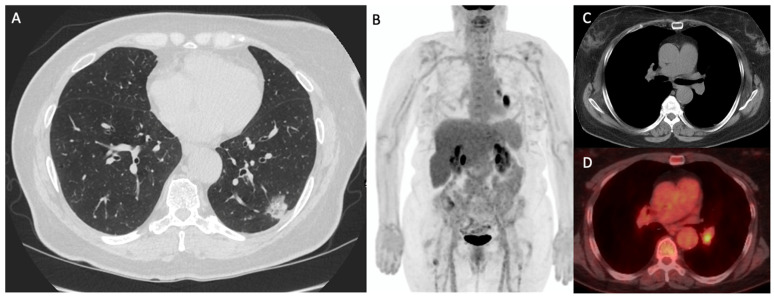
Demonstrates a 62-year-old woman with biopsy-proven left lung adenocarcinoma. (A) Axial CT showing part-solid pulmonary nodule in the left lower lobe, with biopsy demonstrating adenocarcinoma (Stage: T1N1M0). (B) MIP, (C) CT and (D) fused ^18^F FDG-PET/CT maximum intensity projection showing radiotracer avid lung malignancy and uptake in a left hilar node, indeterminate on prior CT, and confirmed as nodal metastasis on subsequent biopsy.
